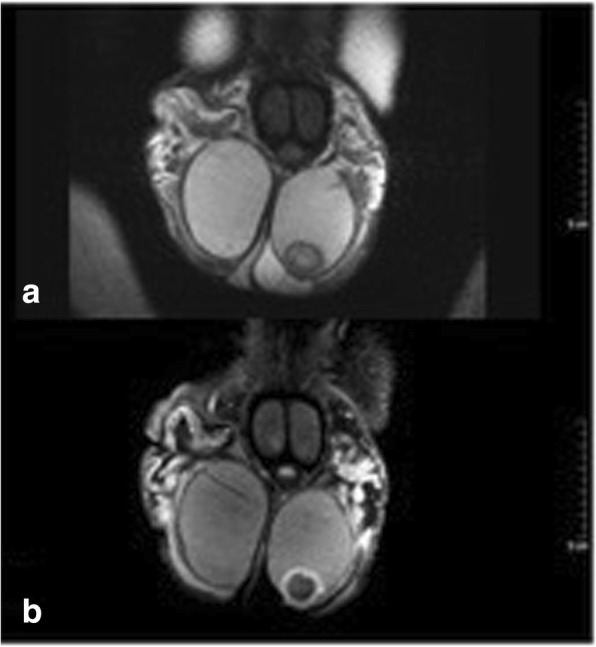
a Patient (adult) with complex Testicular epidermoid cyst, scrotal MRI, 1.5 Tesla, surface coil, coronar section, T1 weighted imaging without contrast material: sharply demarcated roundish cystic lesion at the caudal pole of the left testis. b Patient (adult) with complex Testicular epidermoid cyst, scrotal MRI, 1.5 Tesla, surface coil, coronar section, Gadolinium contrast material: roundish intratesticular cyst at the caudal testicular pole. Note the signal enhancement in the rim region of the cyst, no uptake of contrast material in the cyst centre
